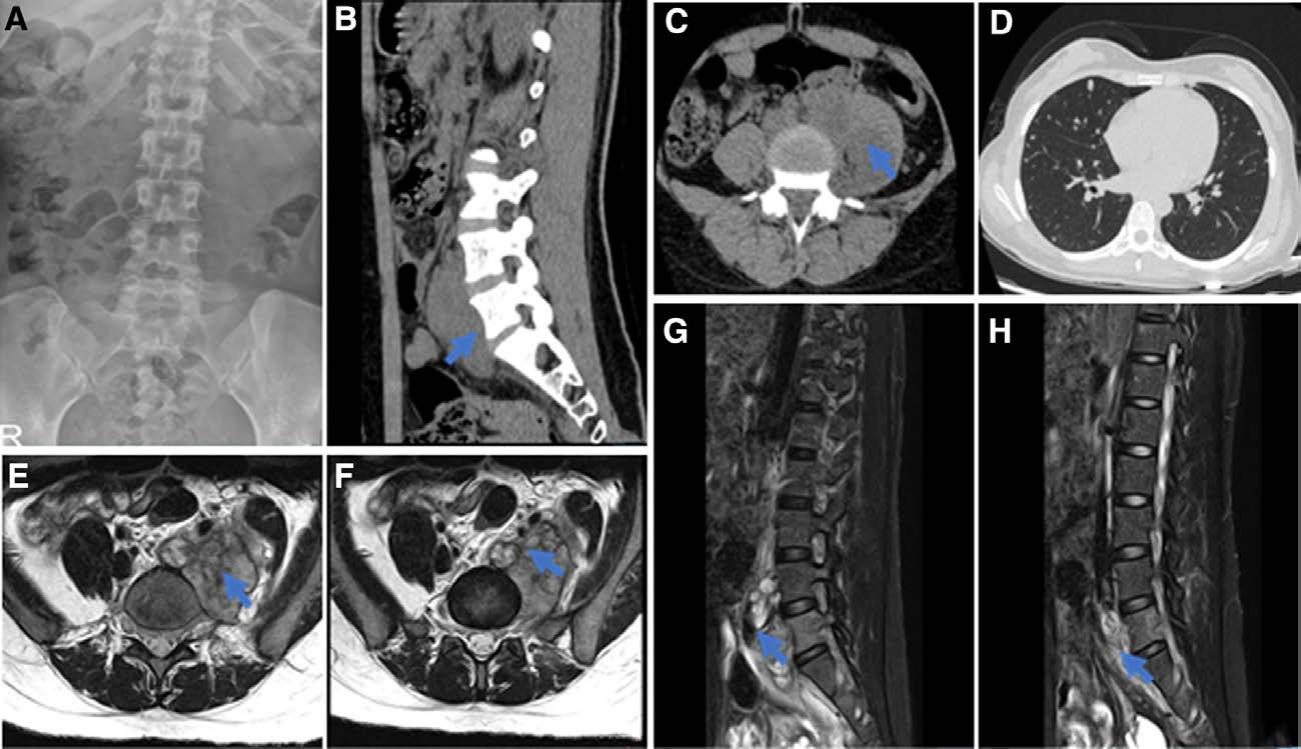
Imaging data before the first surgery. (A) Orthopantomogram of lumbar spine. (B) Sagittal CT image of the lumbar spine. (C) CT transverse section of lumbar spine. (D) Lung CT cross-section. (E, F) lumbar spine MRI cross-sections. (G, H) Lumbar spine MRI sagittal view. CT= computed tomography, MRI = magnetic resonance imaging.
